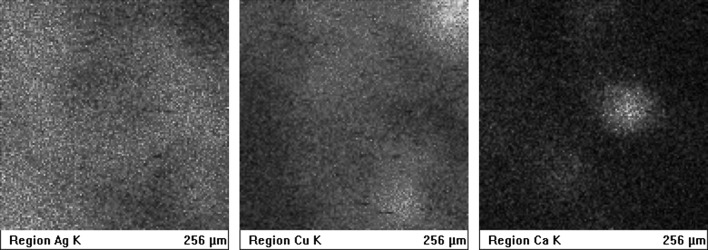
PIXE elemental maps obtained for major elements Ag and Cu, proving a non-uniform distribution of the X-ray signal from the denarius surface. The third map most probably shows an example of surface contamination with Ca
